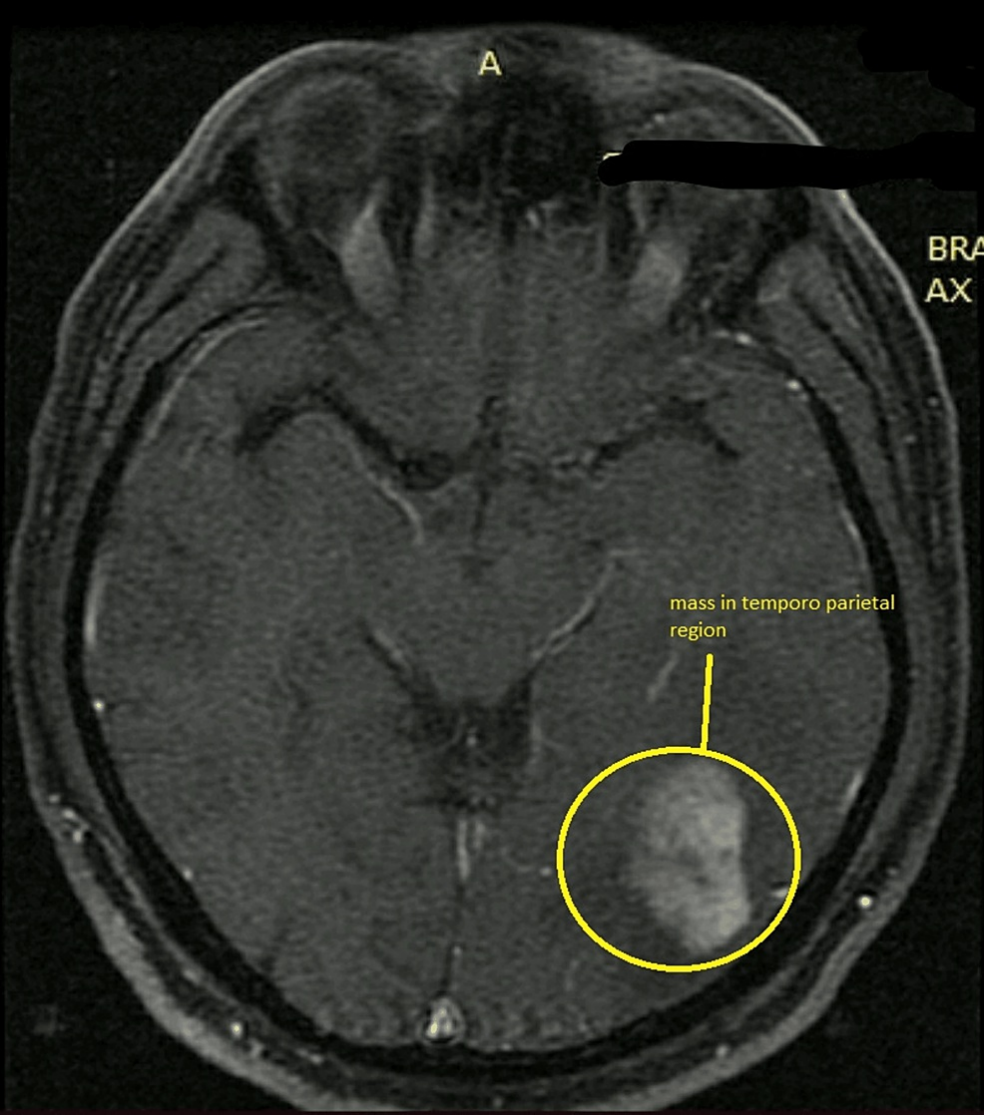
Axial view of the MRI showing lesion in the left temporoparietal region
